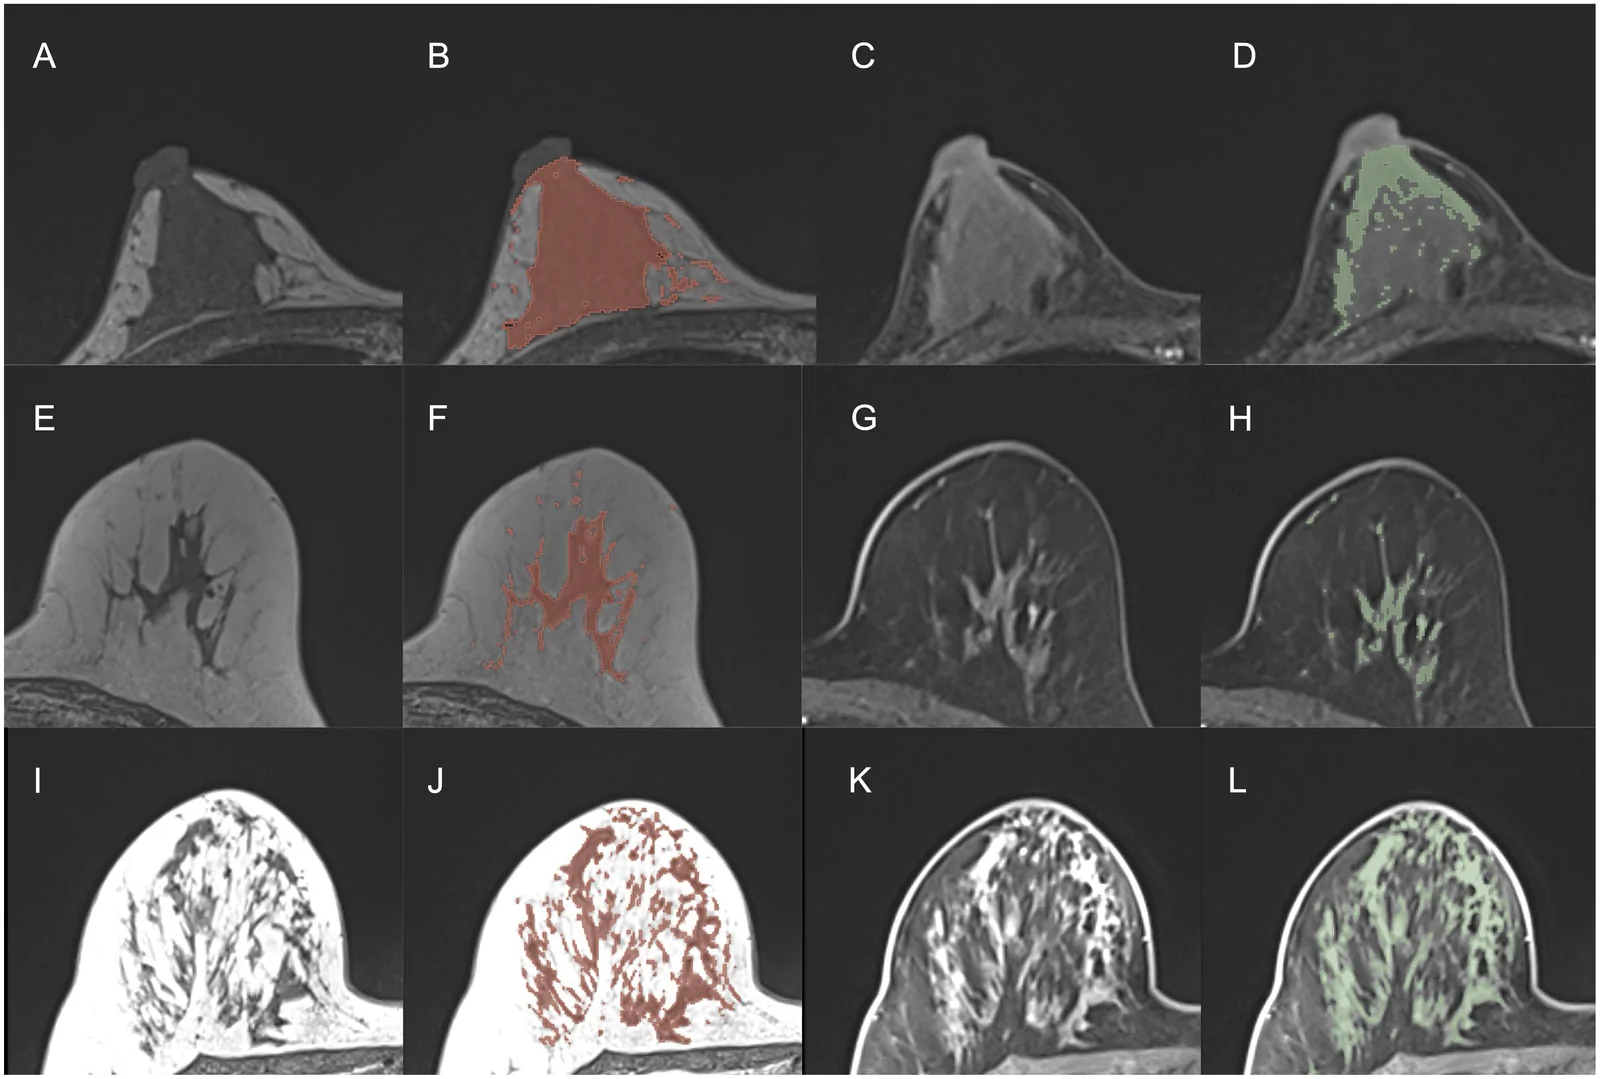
The proportion of background parenchymal enhancement of the patients were 4.1%, 10.5% and 69.1%. (A, E, I) Axial T1-weighted precontrast image with the contralateral breast selected as the region of interest. (B, F, J) Segmentation of fibroglandular tissue (highlighted in red) on the T1-weighted image. (C, G, K) Early postcontrast phase (second dynamic phase) image of the contralateral breast. (D, H, L) Segmentation of enhancing BPE (highlighted in green) on the early postcontrast image.
